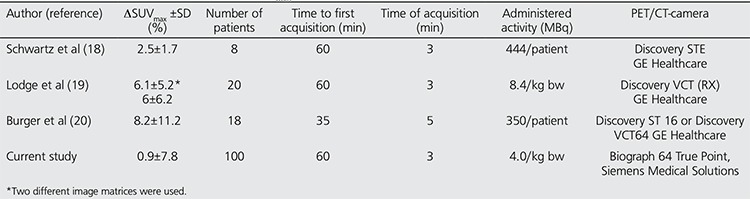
Reports on the repeatability of SUV_max_
